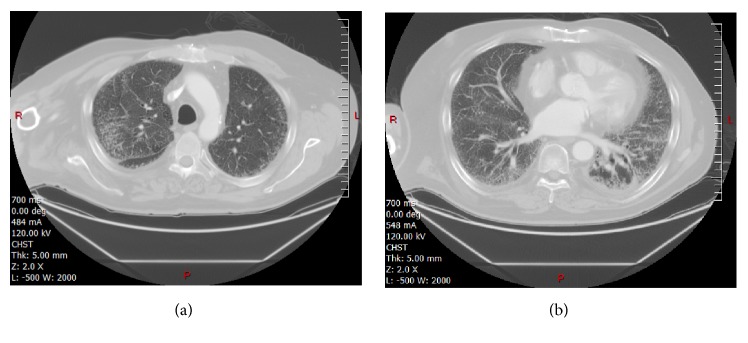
CT images 3 months after the initial presentation of pneumonitis. Images are at approximately the same level as those shown in Figure 6. There has been considerable clearing of the infiltrates and dense consolidation.
